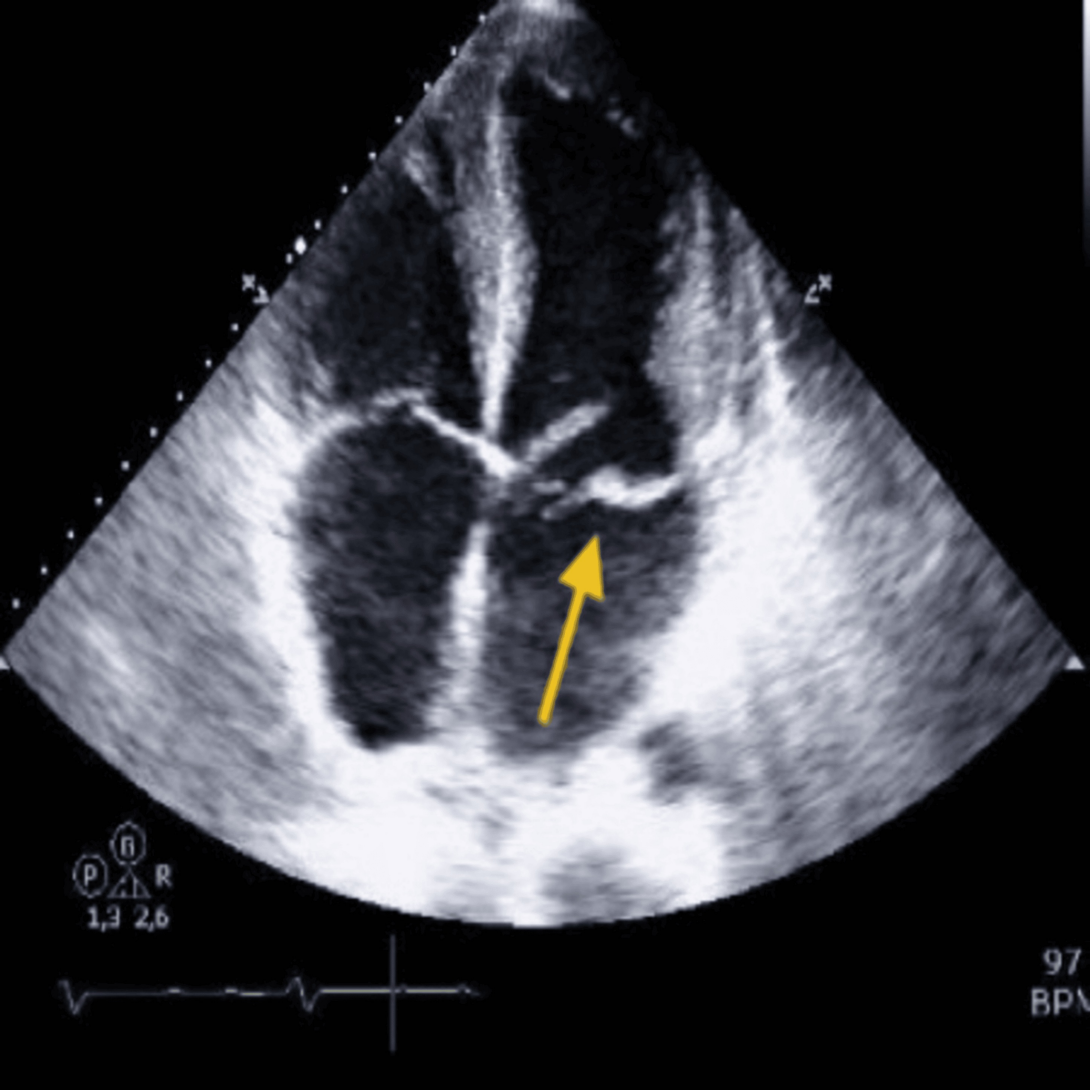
Transthoracic echocardiogram apical four-chamber view revealing a major mitral regurgitation, mild tricuspid regurgitation, and a preserved global systolic function
